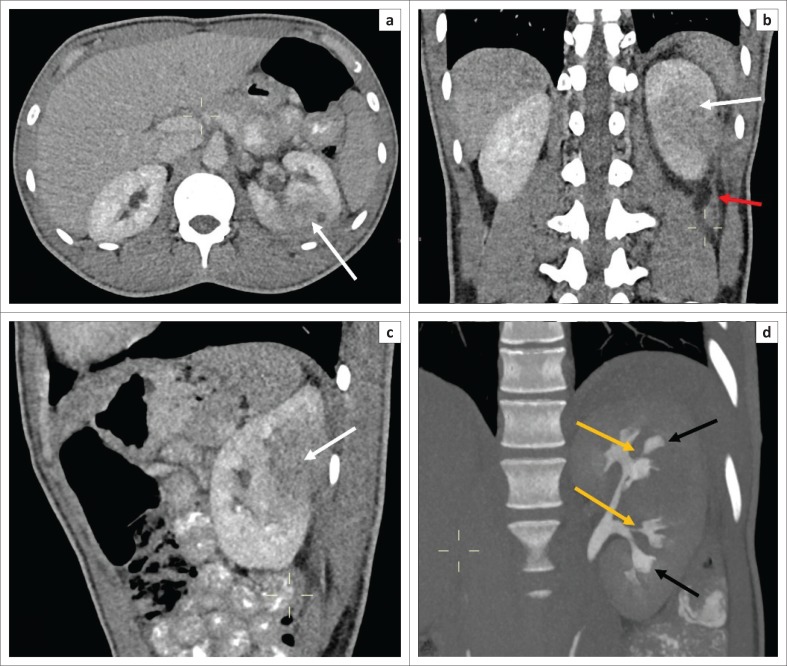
Post-contrast computed tomography (CT) abdomen, venous phase (a – axial, b – coronal, c – sagittal) and d – delay renal phase (MPR coronal) – a, b and c demonstrate a large heterogeneously enhancing left renal mass with a small cystic area (white arrow). (b) There is invasion of the Gerota’s fascia with associated thickening and enhancement (red arrow). (d) There is stenosis of the infundibula (yellow arrows) with slight focal caliectasis and distortion of the calices (black arrows).
